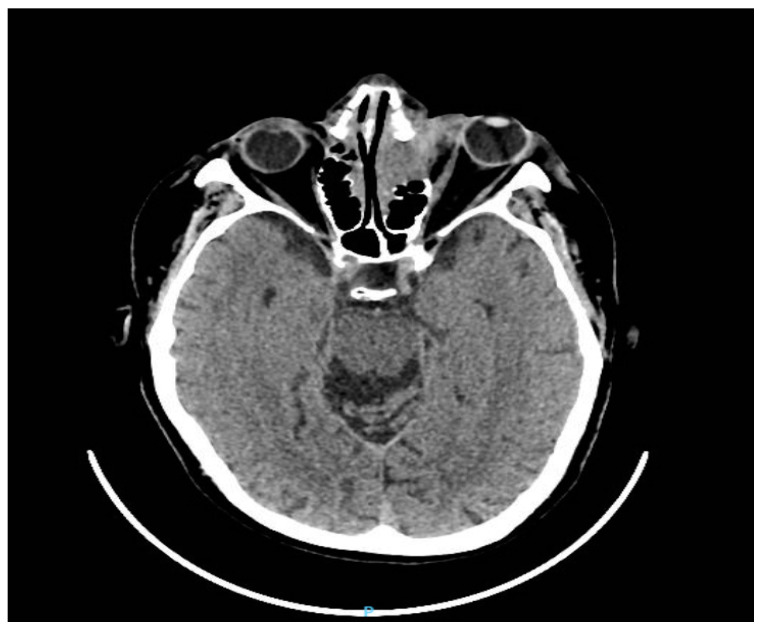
Computed tomography of the brain and orbit (axial planes) showed a front-ethmoid tissue mass with bone destruction and regional invasion with the left orbital extension in contact with the eyeball and left frontal intracranial extension of approximately 3.8/4/4.2 cm. The patient’s treatment began with maximal topical anti-glaucoma eye drops (topical beta-blockers, alpha-agonists, and carbonic anhydrase inhibitors). The patient’s care was subsequently co-managed with the oncological team. He started radiotherapy sessions according to the Intensity Modulated Radiation Therapy (IMRT)) scheme with a total dose of 30 Gy in 15 fractions given in 3 weeks and 2Gy/fraction. The irradiation was done with a thermoplastic mask after a CT planning where the tumor mass and adjacent structures were contoured (red color). Radiation doses delivered to normal anatomical structures (optic nerves, the lens) were within parameters (Figure 4).
